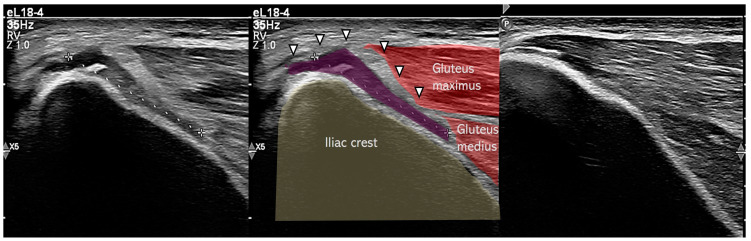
Sagittal ultrasound scans of posterior iliac crest in Case 2: (left) injured side, (middle) annotated scan highlighting periosteal detachment (arrowhead), and (right) uninjured side for comparison.
